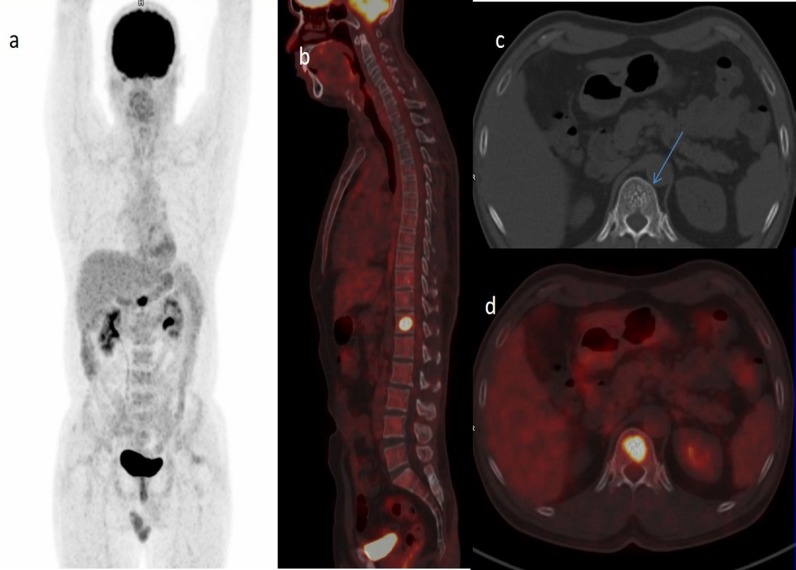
a, b and d) showing FDG avid lesion in T12 vertebra, c) showing classical polka dotted appearance of plain CT images
